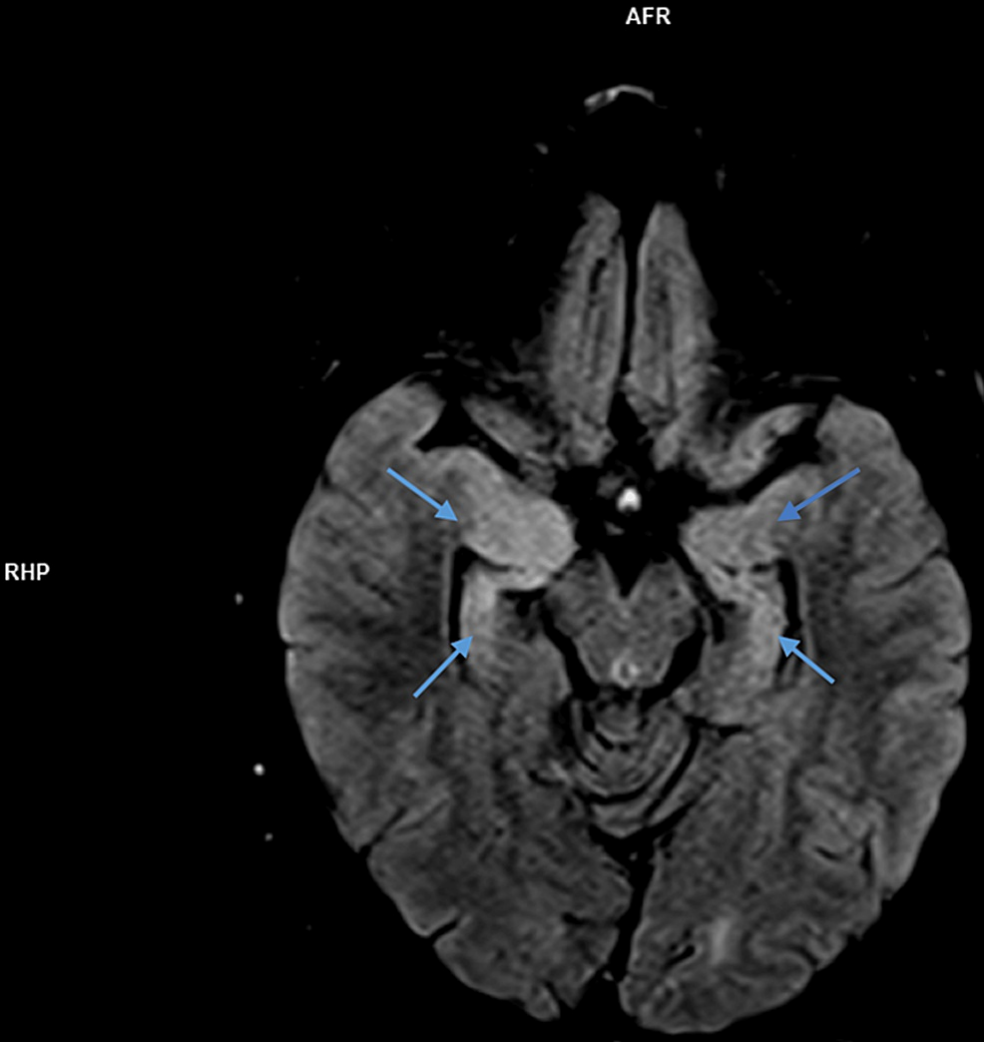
Brain MRI showing bilateral hyperintensities in the temporal lobes
